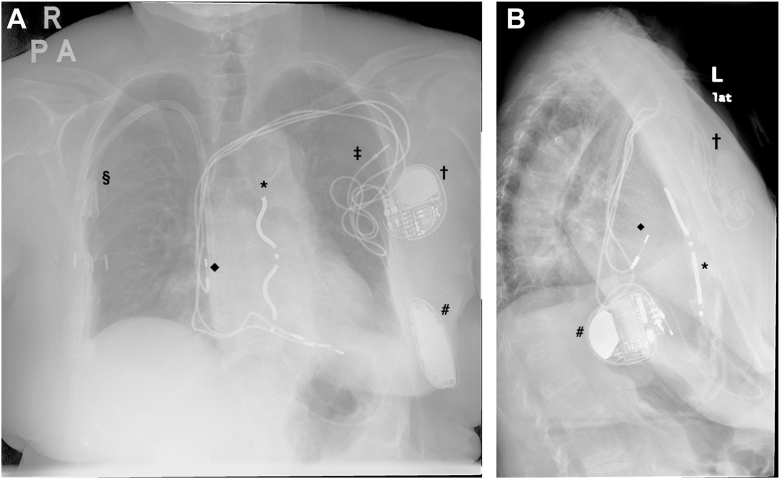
Post-EV-ICD implant chest radiograph. Posteroanterior (A) and lateral view (B) showing the position of the atrial lead of the TV-DCPPM in relation to the Epsila EV lead. ∗ = Epsila EV lead. # = EV-ICD generator. † = TV-DCPPM generator. ‡ = abandoned ventricular lead of TV-DCPPM. ◆ = atrial lead of TV-DCPPM. § = permanent dialysis catheter. EV = extravascular; EV-ICD = extravascular implantable cardioverter-defibrillator; TV-DCPPM = transvenous dual-chamber permanent pacemaker.
